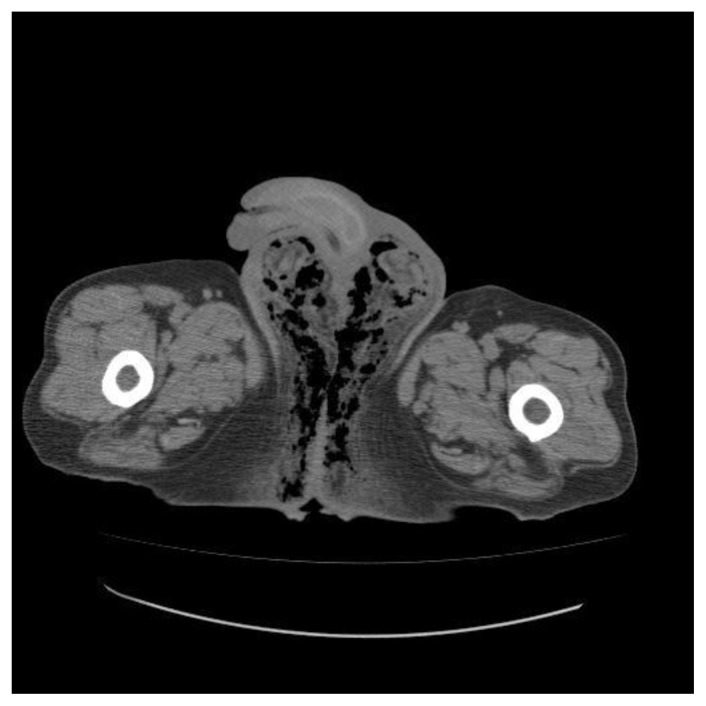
CT scan of a patient with FG showing emphysematous gangrene of perineum and scrotum (A). two additional parameters, such as age and extent of the disease.^13^
